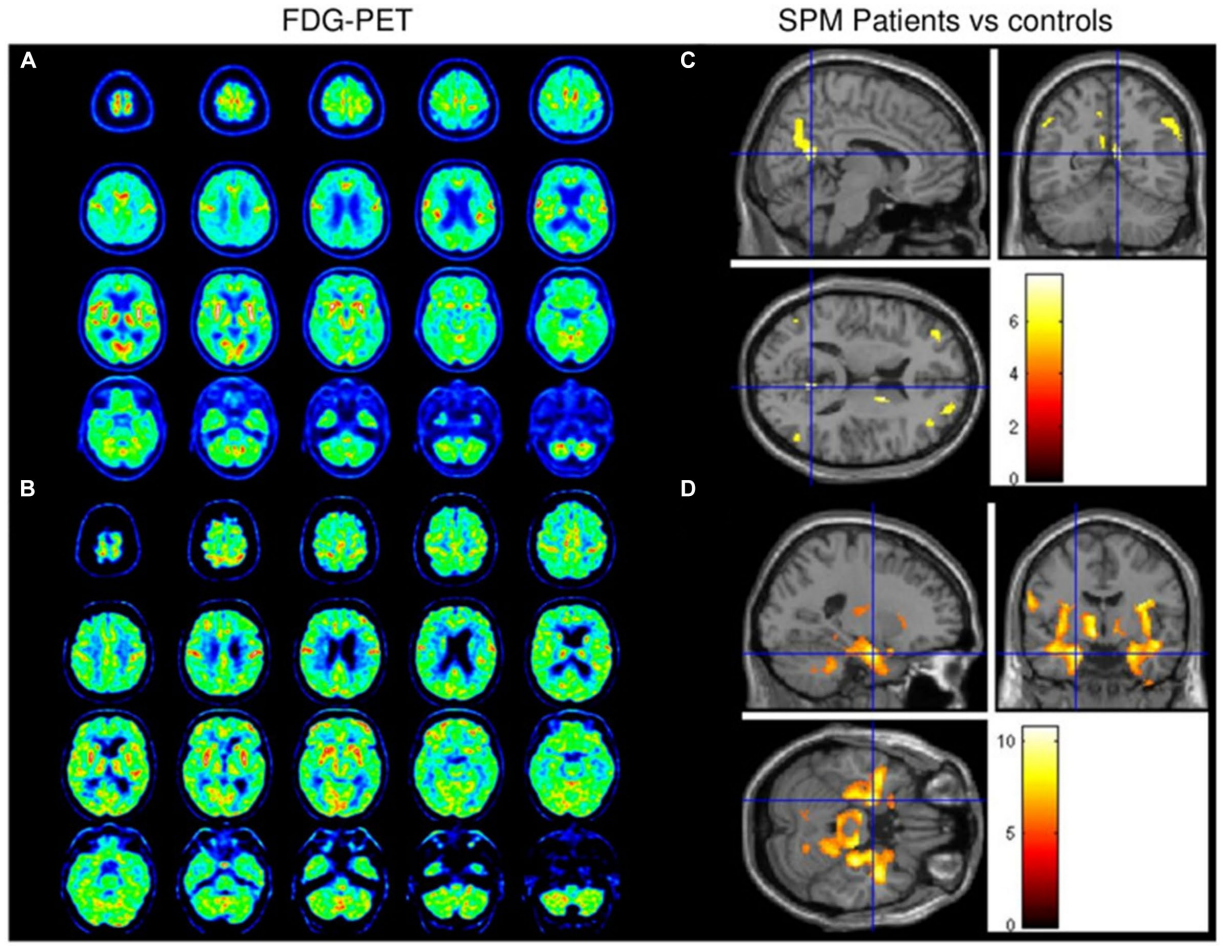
Brain FDG-PET data from patients 1 (A) and 2 (B) revealed diffuse cortical hypometabolism with relatively preserved metabolism in the sensorimotor cortex. Hypometabolism was observed in the bilateral dorsolateral prefrontal cortex, right caudate nucleus, and bilateral posterior parietal cortex (C). Marked relative hypermetabolism was observed in the bilateral middle temporal cortex, basal ganglia, brainstem, and cerebellum (D). Reprinted with permission, Morassi et al. SARS-CoV-2-related encephalitis with prominent parkinsonism: clinical and FDG-PET correlates in two patients (79).
